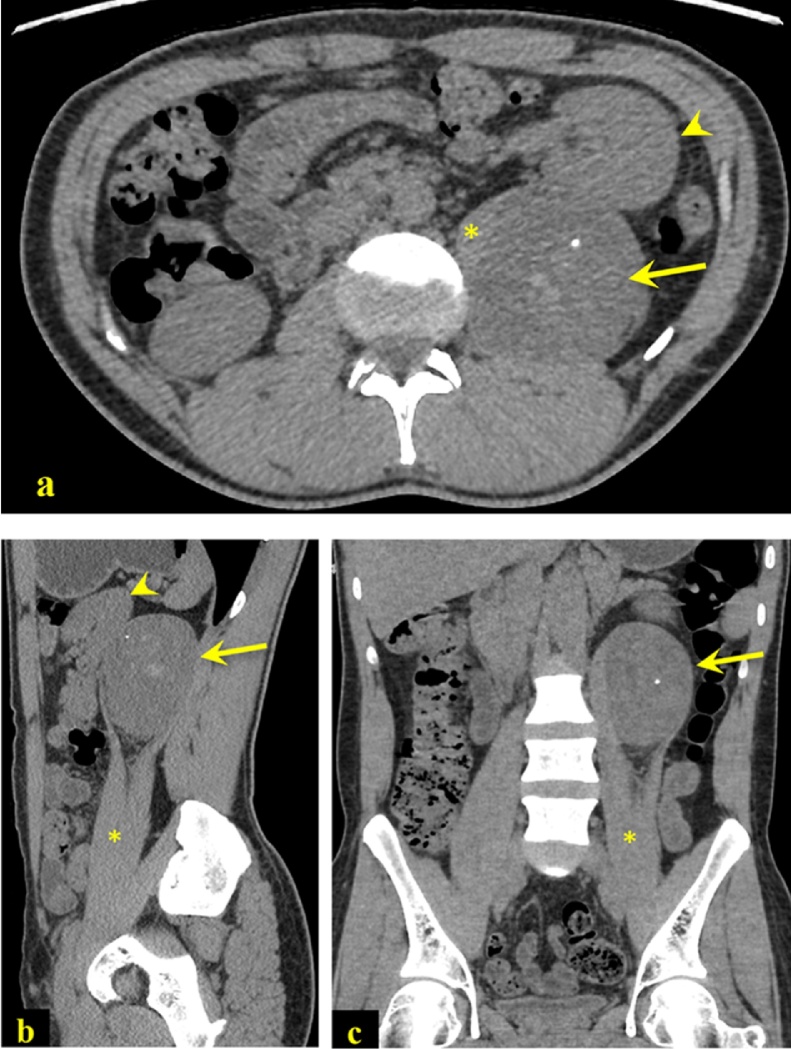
Axial (1a), sagittal (1b), and coronal (1c) CT scan of the abdomen without IV contrast obtained initially for renal colic. There is a well-defined oval paraspinal soft tissue mass (arrow) within the left psoas muscle (asterisk). The mass is heterogeneously hyperdense with foci of calcifications. The left kidney is displaced anteriorly (arrowhead).
